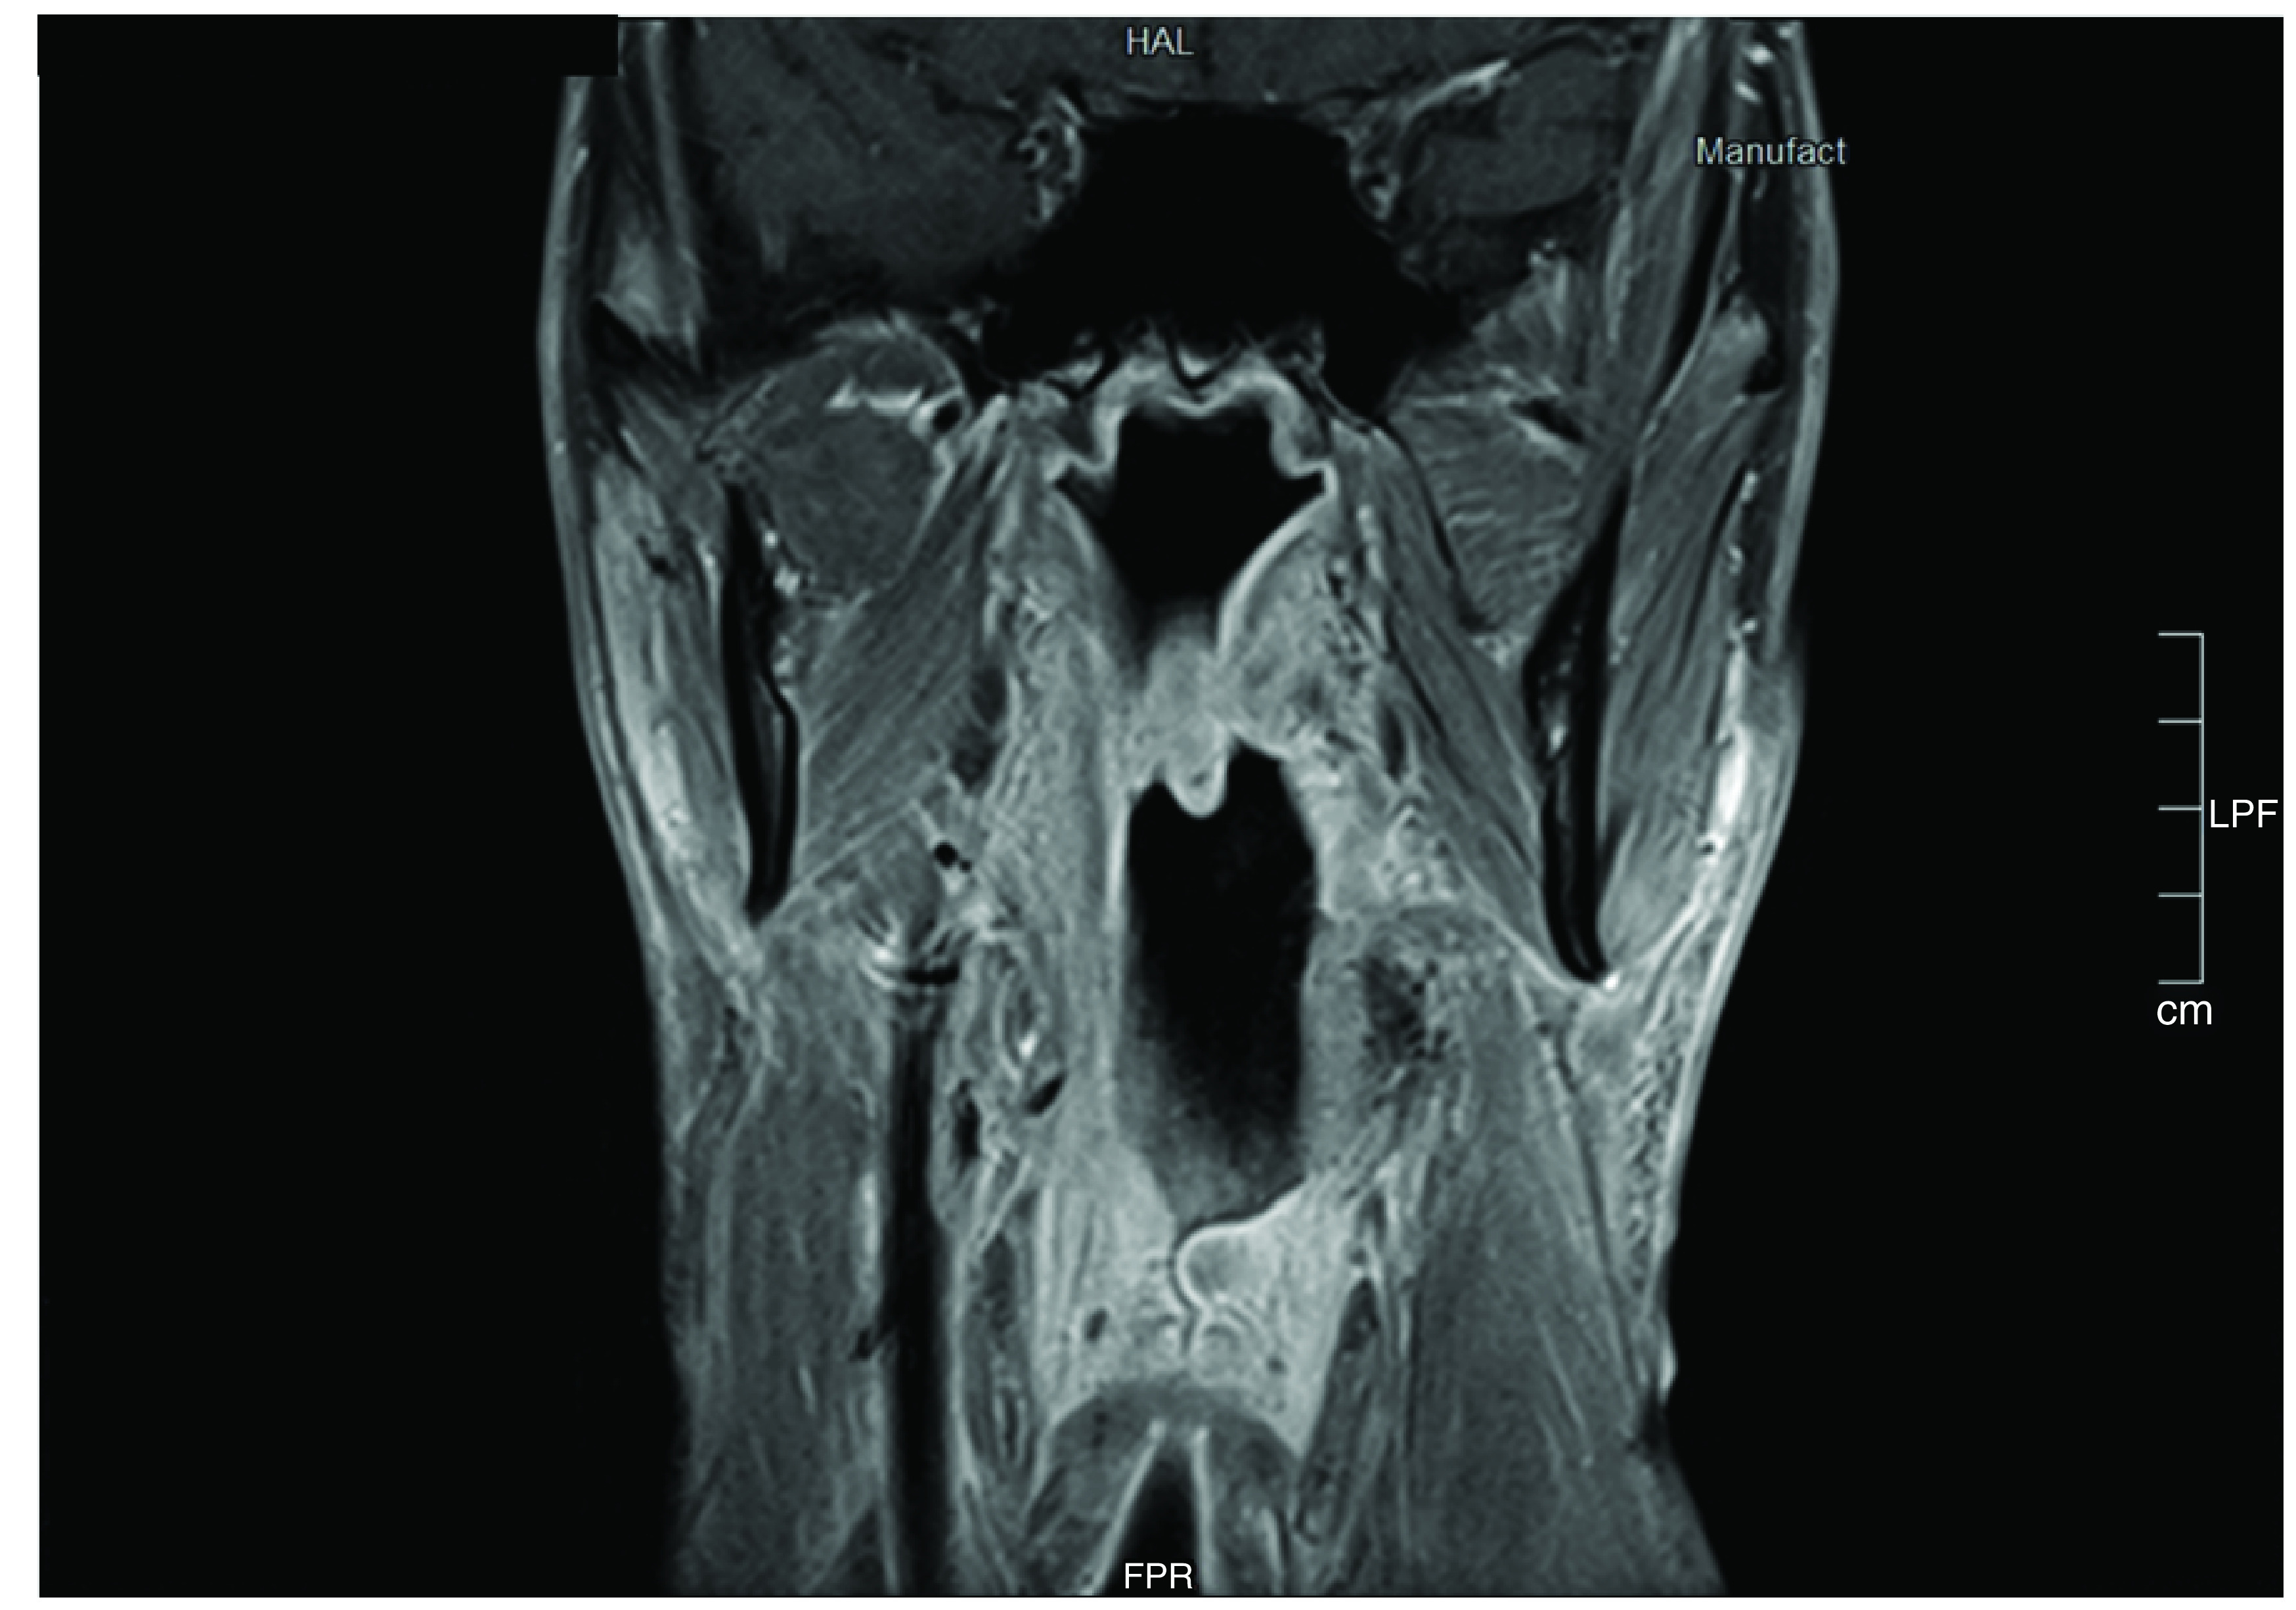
A 5-year postoperative MRI scan of the pharynx.
